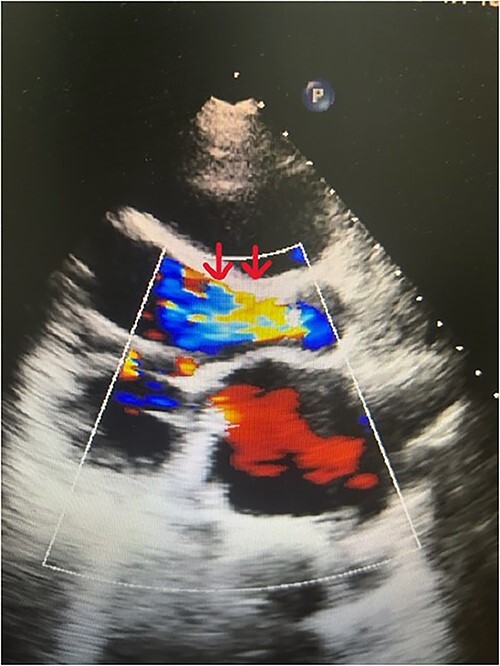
Doppler echocardiography shows severe AR (red arrows).
